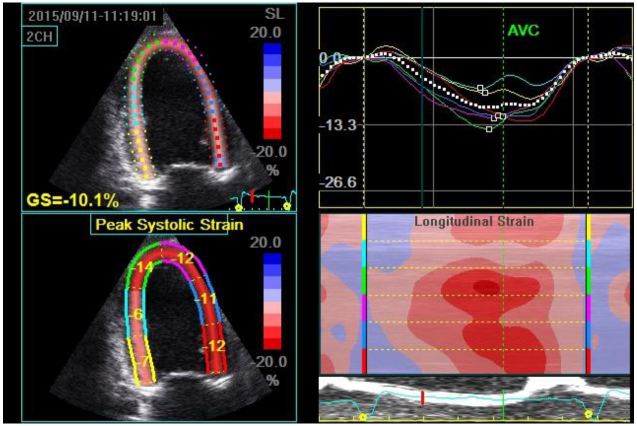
Quad display of an apical 2-chamber image demonstrating three different ways to depict left ventricular (LV) longitudinal strain. Images are taken from a patient with a history of heart failure with preserved ejection fraction. Top left image is a 2D depiction showing the color-coding for each LV segment and the global longitudinal strain in the 2-chamber plane (GS=−10.2%); bottom left image displays the strain for each of the 6 LV segments in the 2-chamber plane; bottom right shows the anatomical M-mode display for the 2-chamber plane with each LV segment color coded on the y-axis and the instantaneous strain being depicted using deeper red hues to represent more negative strain and deeper blue hues to represent more positive strain; top right displays strain (y-axis) plotted over time (x-axis) for each LV segment with a color-coded linear graphical display.
